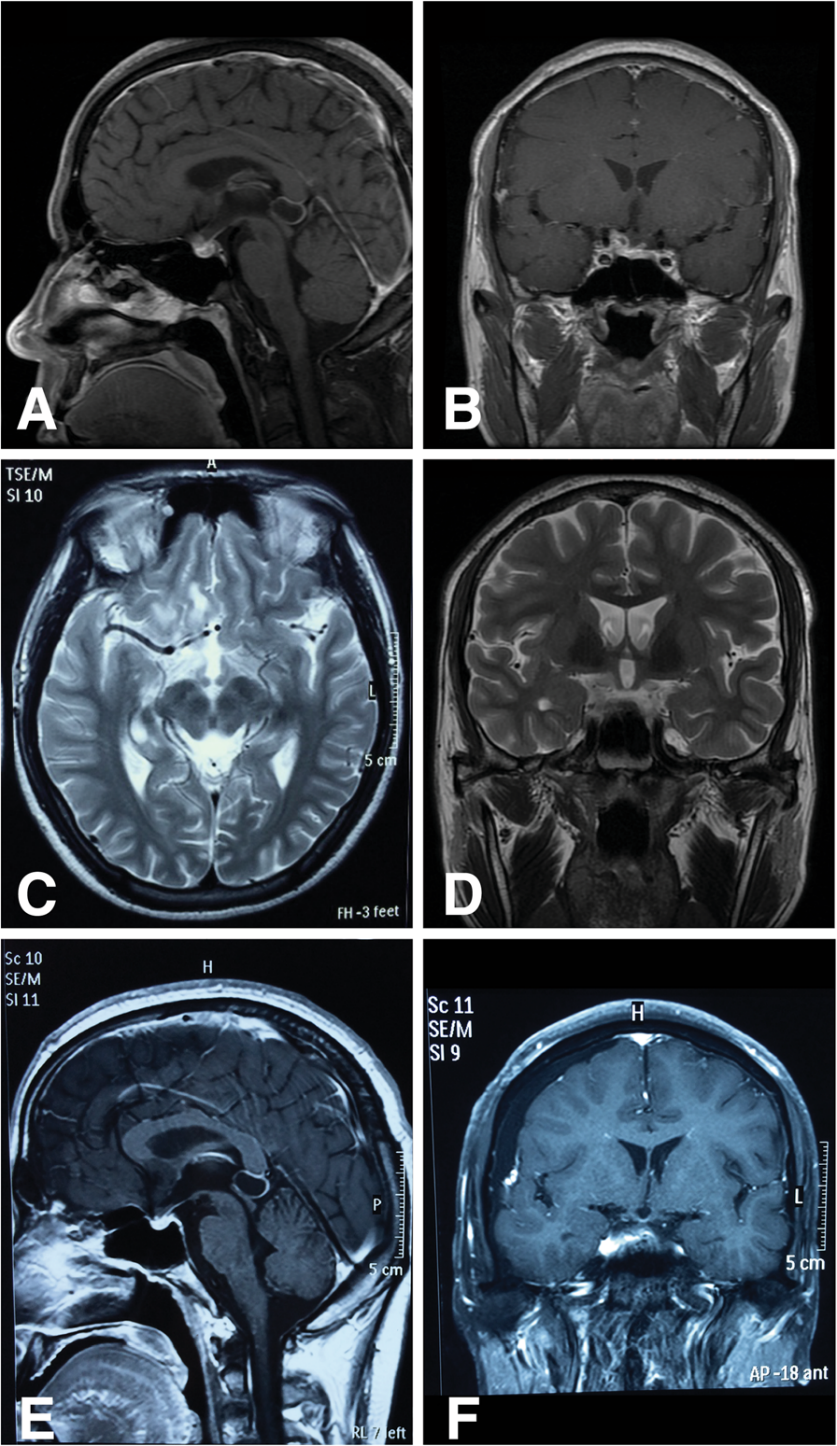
Magnetic resonance imaging (MRI) scans at recurrence and after biopsy. a Enhanced sagittal T1 sequence: scans after the recurrence of headache in Sept. 2014 showed an enlarged sella with a slightly sunken bottom. The pituitary was enlarged with a height of 1.15 cm and showed a heterogeneous signal, with a patchy, short T1 signal and an abnormal, long T2 signal. The pituitary stalk was shortened and thickened. b Enhanced coronal T1 sequence: a new lesion was detected after recurrence. There was nodular enhancement with abnormal T1 and T2 signals on the right side of the suprasellar region; the lesion was invading the pituitary stalk, infundibulum, right optic nerve, posterior right basal gyrus rectus of the frontal lobe, anterior perforated substance and extending to the internal carotid artery. c and d Enhanced T2 sequence: cerebral parenchymal edema was detected around the lesion. e Enhanced sagittal T1 sequence: changes were observed in the pituitary after the biopsy and i.v. administration of glucocorticoids. f Enhanced coronal T1 sequence: after the biopsy and i.v. administration of glucocorticoids, the parenchymal edema was significantly reduced
